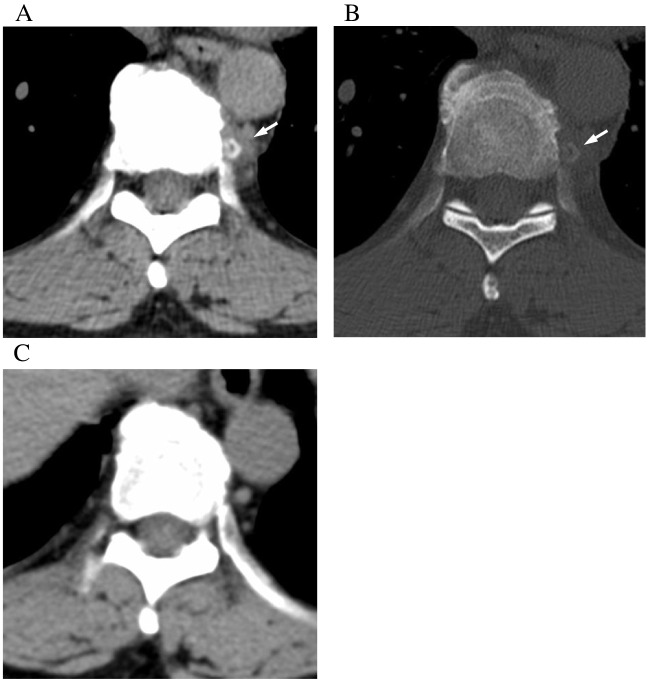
50-year-old women with back pain (Patient #16). A CT image setting shows soft tissue density and central paravertebral calcification on the left side of the vertebral body at the level of the Th 9/10 intervertebral disc (arrows). The paravertebral calcification appears heterogeneous. B On CT images with bone window setting, the paravertebral calcification demonstrates lower attenuation compared to the cortical bone of vertebrae. The CT value measurement indicated that the maximum value of the calcification was 255 HU. C On the CT scan obtained 3 years later, the calcification has resolved
